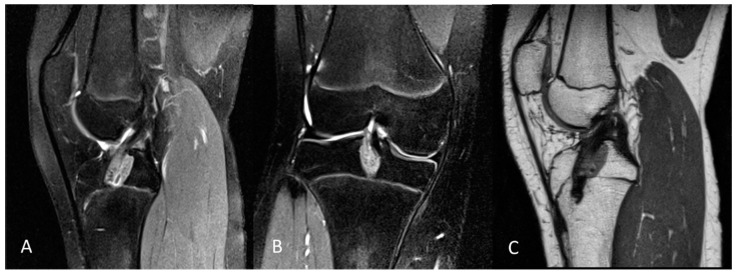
Example of a postoperative 3.0 Tesla knee MRI: (A) sagittal and (B) coronal PD tse fs, and (C) sagittal PD tse of a 14-year-old female patient with open physis and postoperative MRI after ACL reconstruction. PD tse fs (proton density-weighted turbo spin echo sequence with fat saturation), PD tse (proton density-weighted turbo spin echo sequence).
